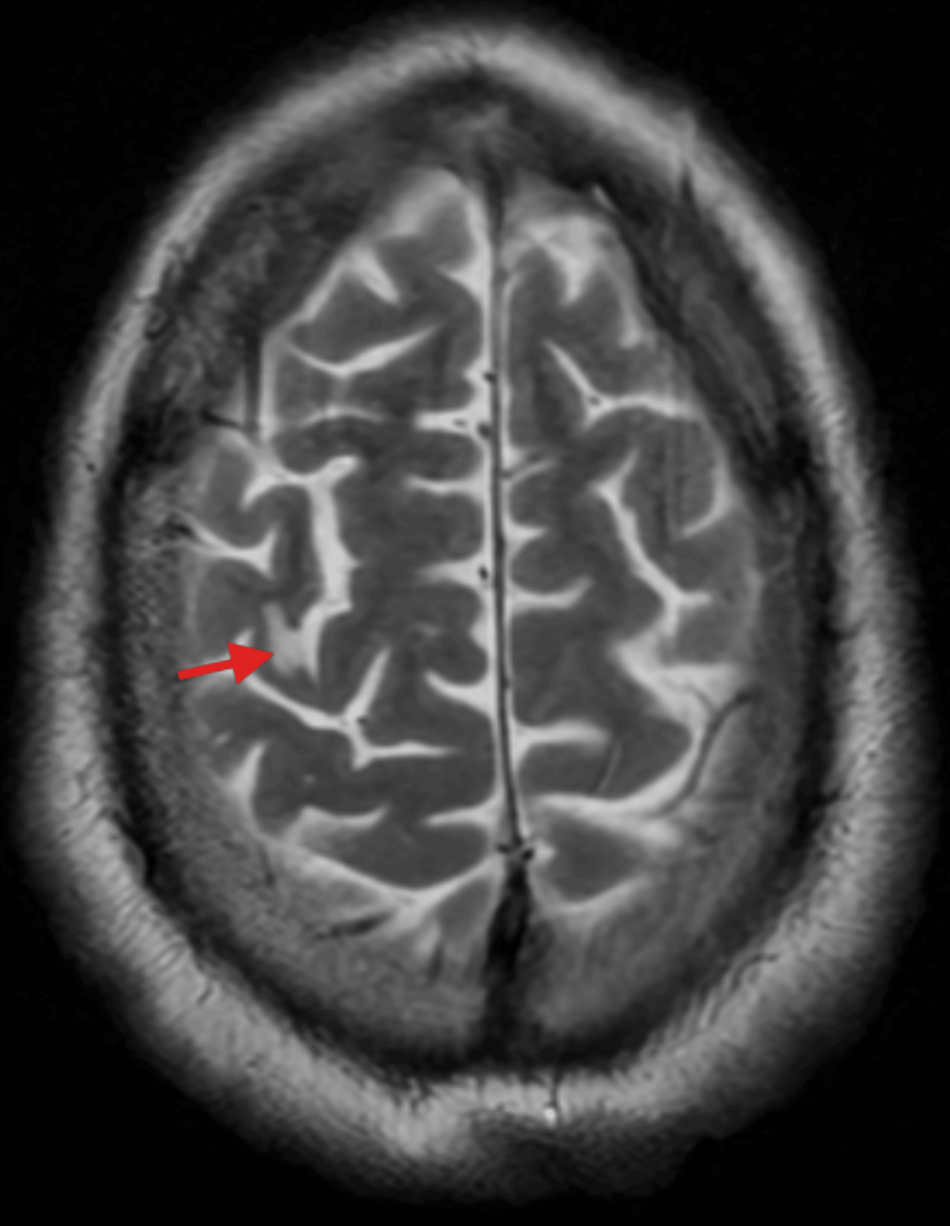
Axial T2-weighted MRI of the brain. The arrow indicates a small, chronic cortical infarct in the right pre-central gyrus (motor strip). No posterior circulation infarct is visible.
